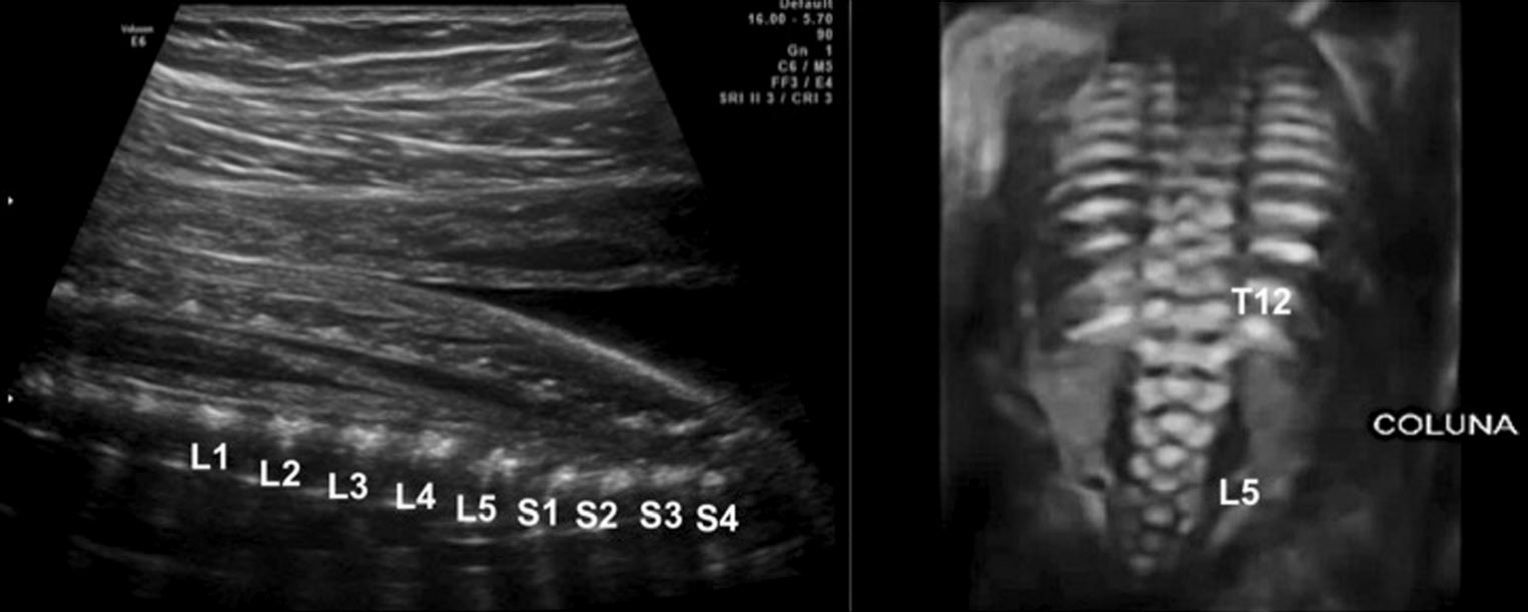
Two-dimensional ultrasonography. Counting of vertebral bodies by determining the 12th thoracic vertebra that corresponds to the last rib
